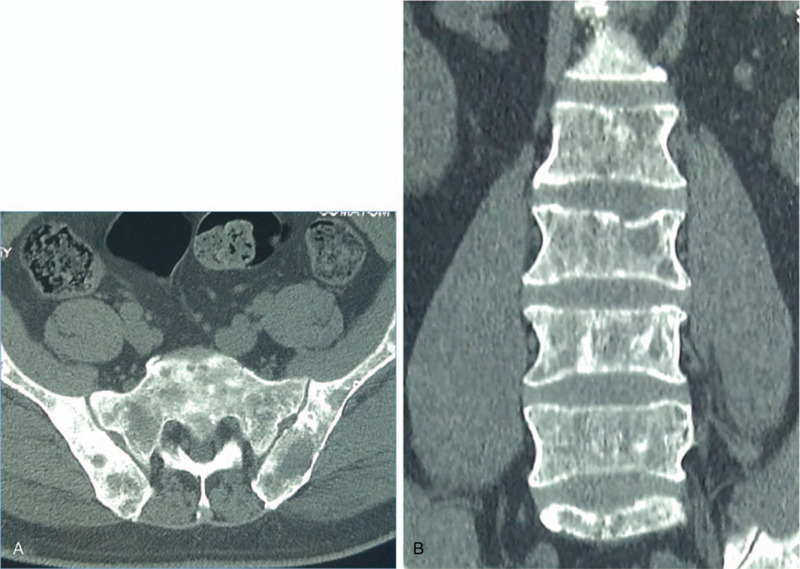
Demonstrating an extensive bone lytic lesion of the whole spine or pelvic bone No tumor in other body soft tissues or organs was discovered by CT (1A for Pelvis CT at horizontal plane, 2A for Lumbar CT at coronal plane).
